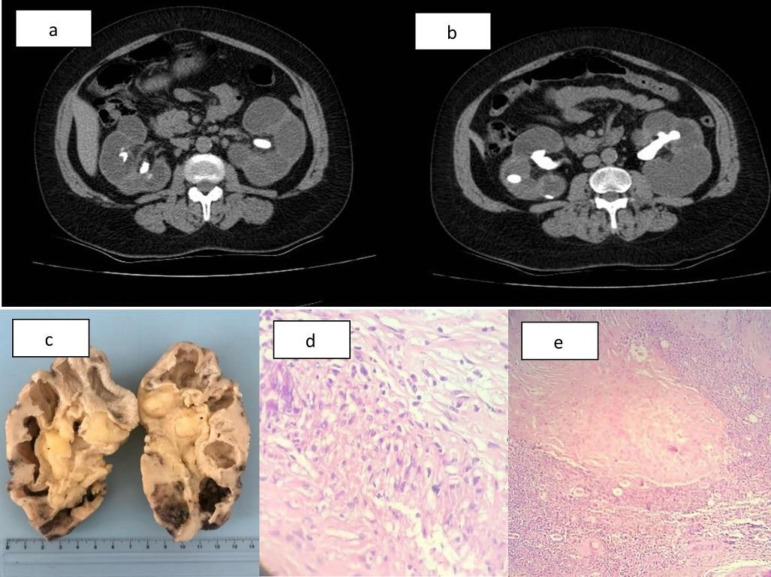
CT scan showing the “bear paw sign” in both kidneys (a) and staghorn
